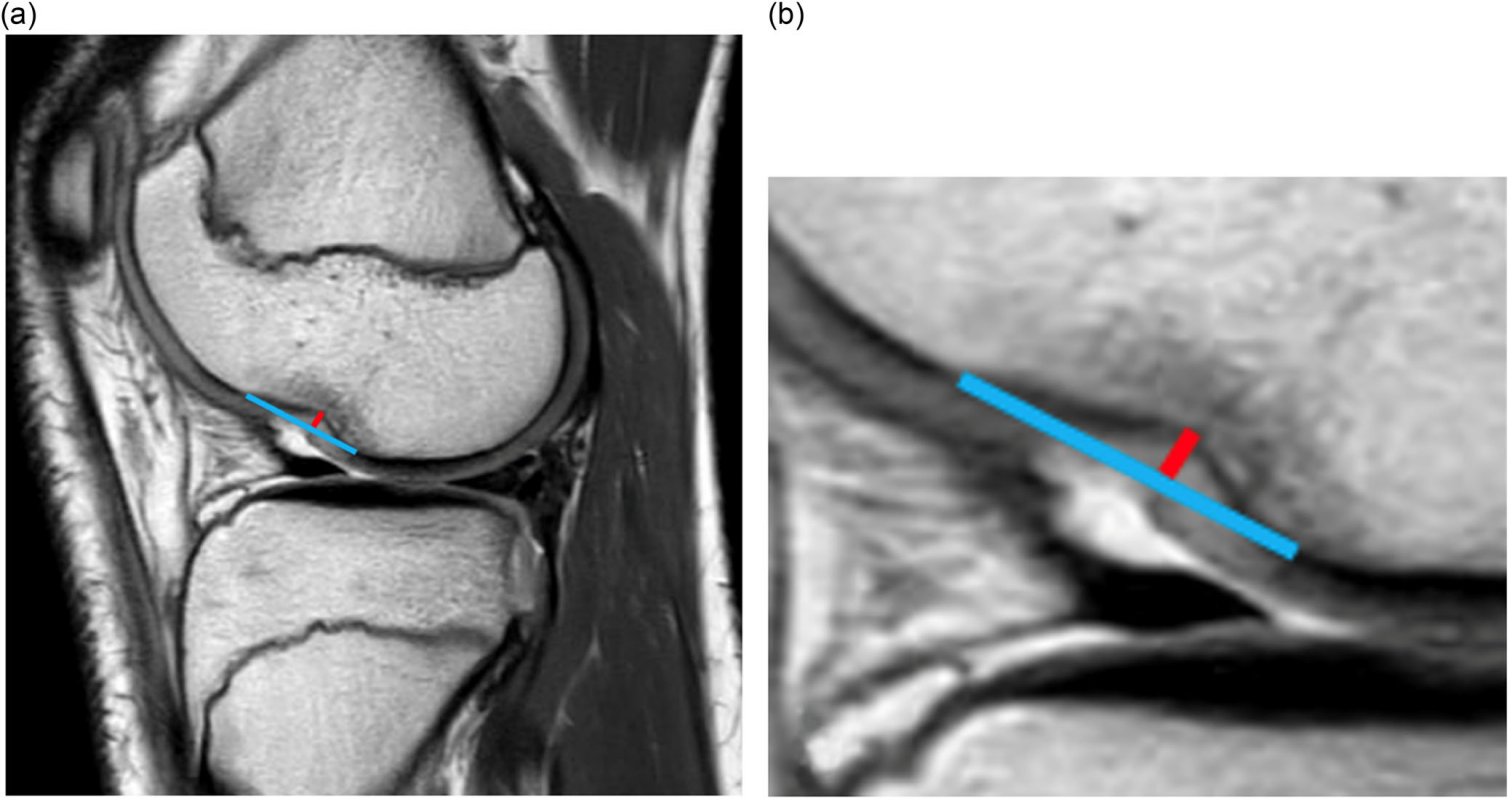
(a) Measurement technique to determine the depth of the deep lateral femoral notch sign (DLFNS) on proton density weighted (PDW)‐ or T1‐weighted sagittal magnetic resonance imaging (MRI). The blue line represents the tangent line connecting the anterior and posterior edges of the impression; the red line indicates the DLFNS depth, defined as the perpendicular distance from the tangent line to the deepest portion of the impression. (b) Zoomed‐in view of (a) to provide a more detailed visualisation of the measurement.
